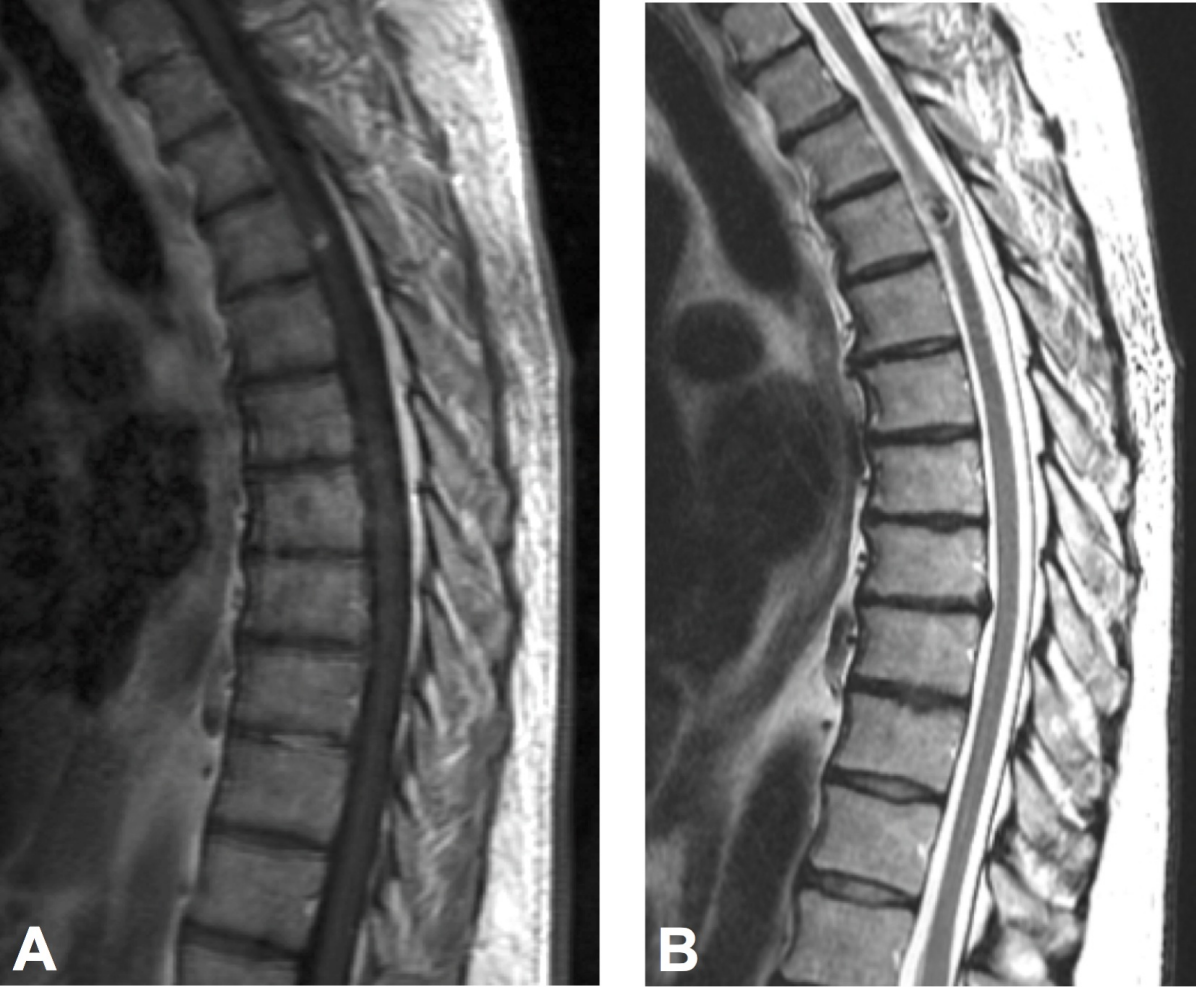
Thoracic spine MRI demonstrating an intramedullary cavernous malformation at the T4 level, Sagittal view in (A) T-1 sequence depicting a high-intense lesion and (B) T-2 sequence with hypointense signal and minimal edema.
